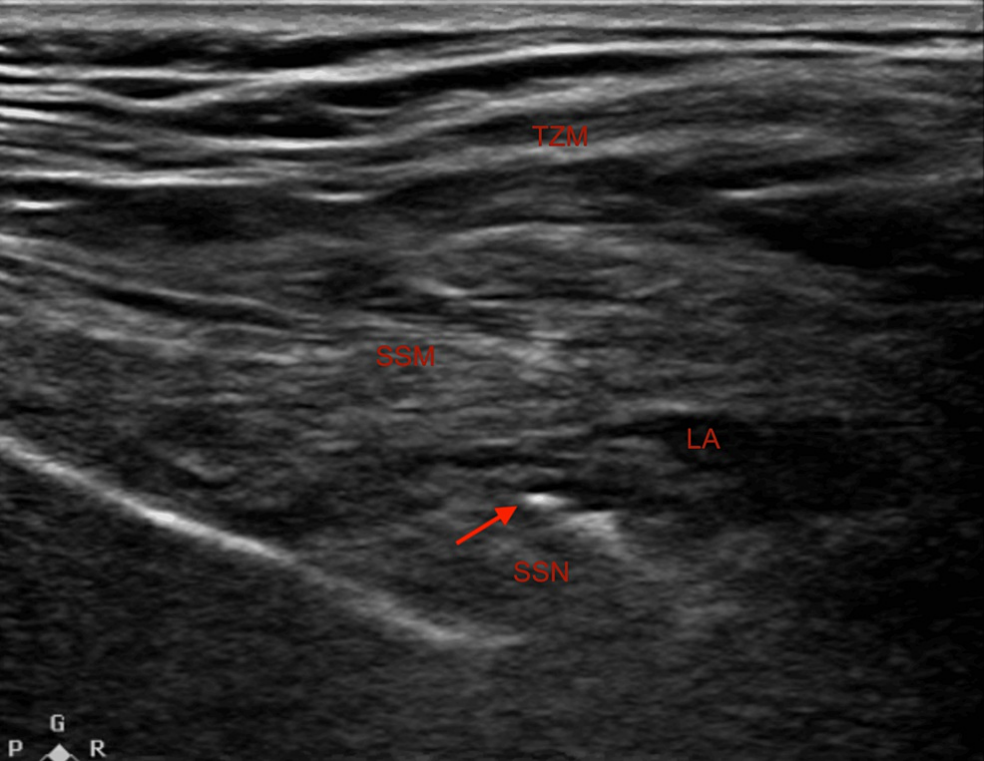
Ultrasound-guided placement for the suprascapular nerve block. TZM: trapezius muscle; SSM: supraspinatus muscle; LA: local anesthetic; SSN: suprascapular nerve; Arrow: needle tip
